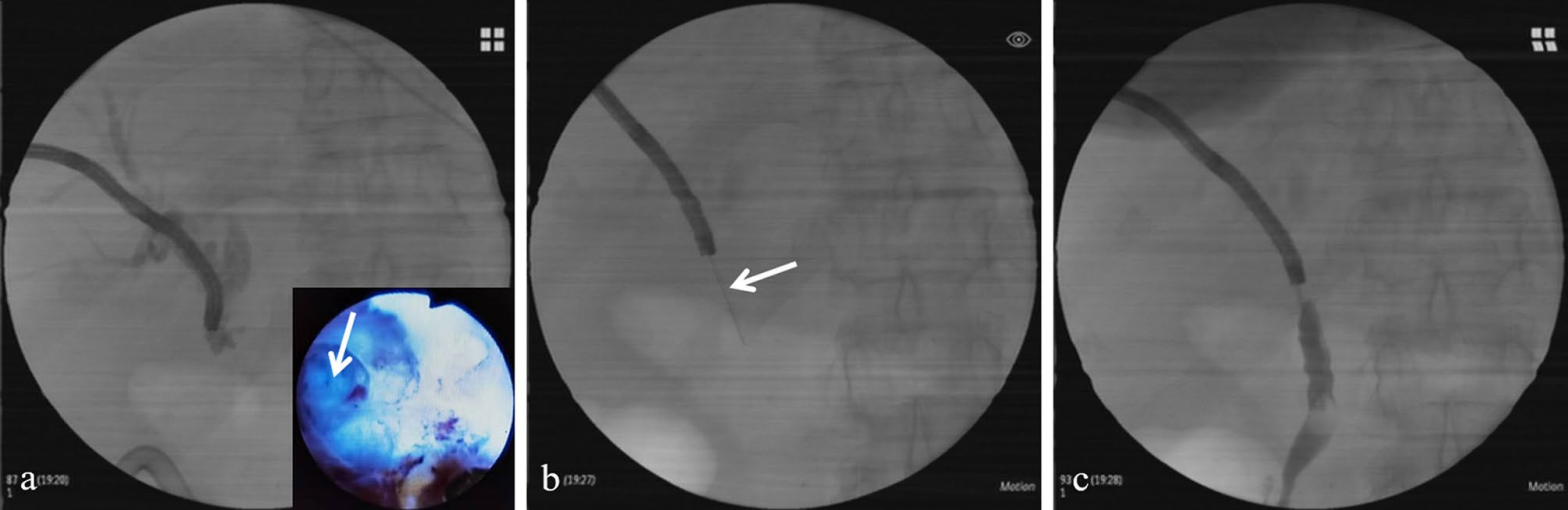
PTCS-assisted puncture of the complete biliary anastomotic strictures using the hard tip of the guidewire. (a) The bilio-biliary anastomosis is an eccentric occlusion (arrow) under PTCS; although contrast agent was injected, the distal duct does not become visible. (b) Hard-tip puncture (arrow) of the stricture is conducted under PTCS. (c) Recanalization is confirmed by the visible distal duct through radiography. *PTCS* percutaneous transhepatic cholangioscopy.
